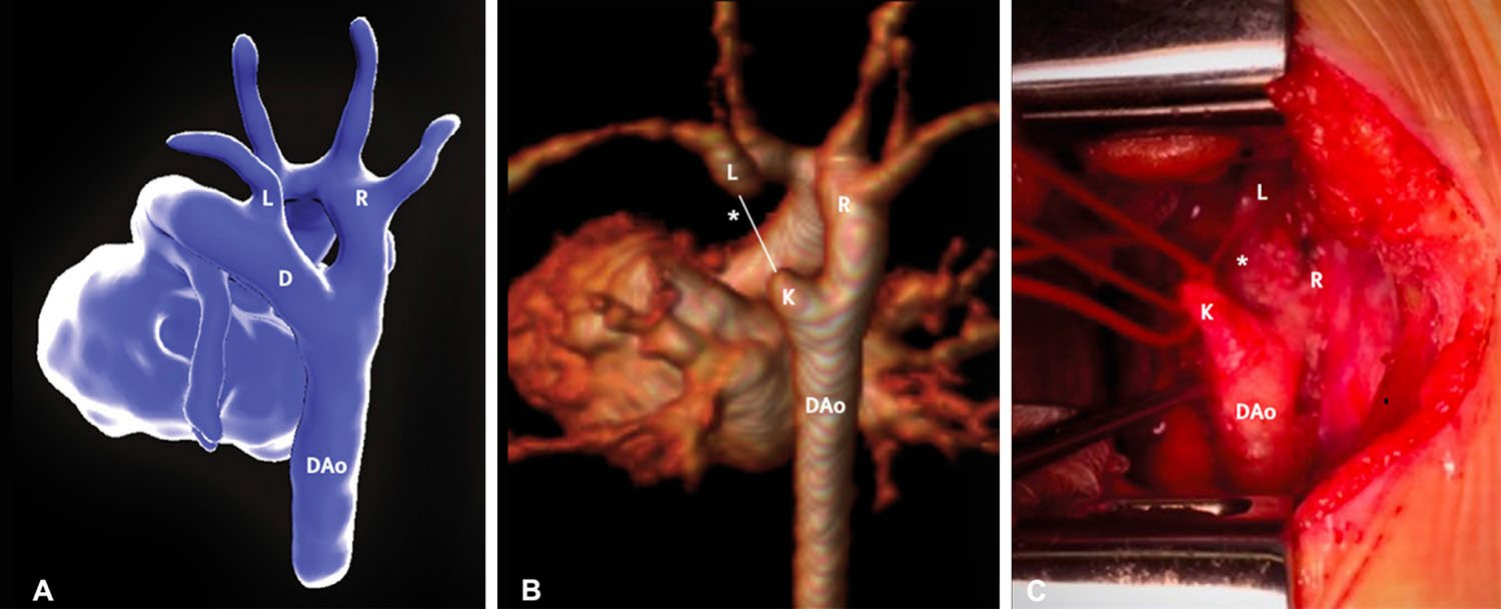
Motion-corrected MRI data from a fetus with double aortic arch at 32 weeks (A). Shown are the descending aorta (DAo), arterial duct (D), and left (L) and right (R) aortic arches. At 2 months postnatal age, contrast-enhanced MRI could show a right-sided arch (B); however, a ligamentous remnant of the left arch was predicted on the basis of the fetal MRI findings (asterisk); this finding was confirmed at surgery (C). The distal remnant of the arterial duct—analogous to the diverticulum of Kommerell—is also seen (K). (From: Lloyd D, et al. Lancet 2019; 393: 1619–27, with permission from Elsevier) [31]
